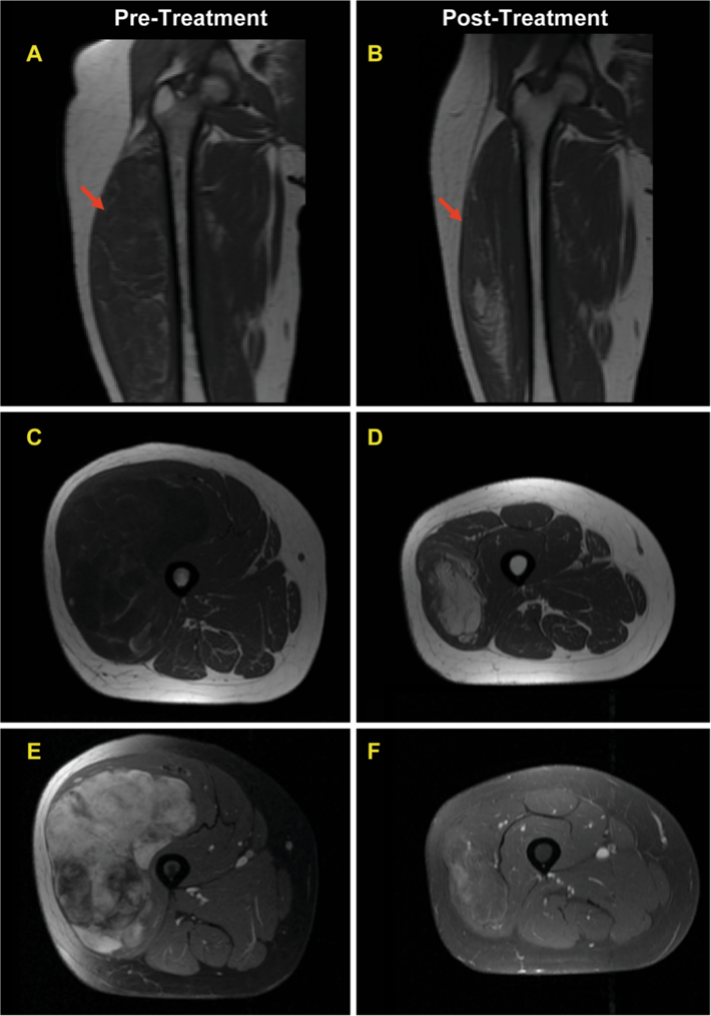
MRI findings in a case of myxoid liposarcoma which developed extensive adipocytic maturation during treatment. T1 weighted coronal right thigh (A) pre-treatment and (B) post treatment demonstrating a marked decrease in tumor size. T1 weighted axial image of right thigh (C) pre-treatment and (D) post treatment demonstrate an increase in signal characteristic of mature adipose tissue content (>50%) with reduction in size. T1 weighted axial with contrast media and fat saturation (E) pre-treatment showing large mass with extensive vascular enhancement. (F) Post treatment scans show a reduction in size of mass and a decrease in vascular enhancement greater than 50%.
